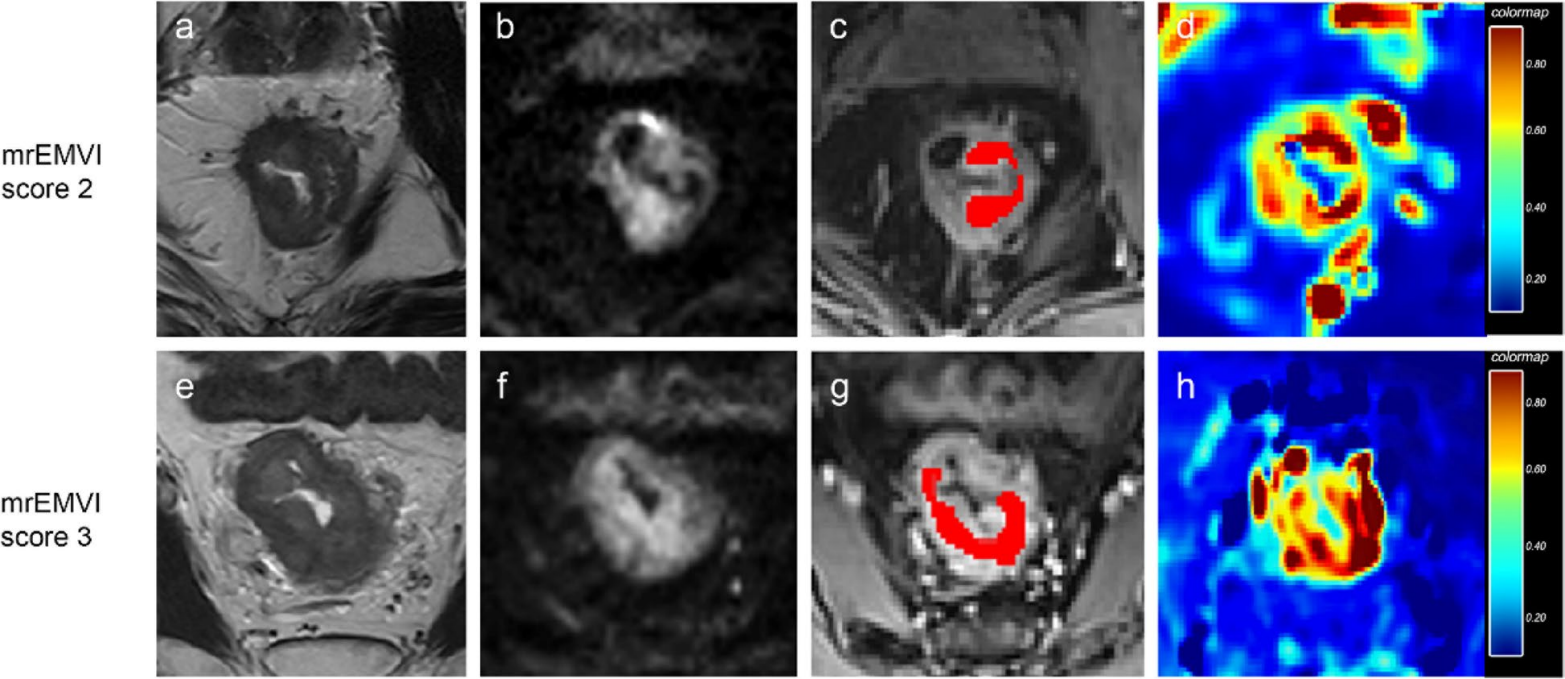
Image analysis of two male patients with mrEMVI score 2 and mrEMVI score 3 respectively. (a, e) Oblique axial T2-weighted (T2W) images. (b, f) Diffusion-weighted (DW) images at b = 1000 s/mm^2^. (c, g) Regions of interest (ROI) result on DCE-MRI. (d, h) Corresponding *K*^*trans*^ maps
